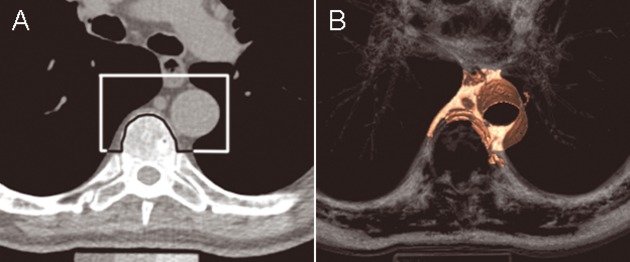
(A) Periaortic fat in an axial CT image (upper boundary) with (B) corresponding 3D reconstruction. Used with permission from Fox et al.^8^
